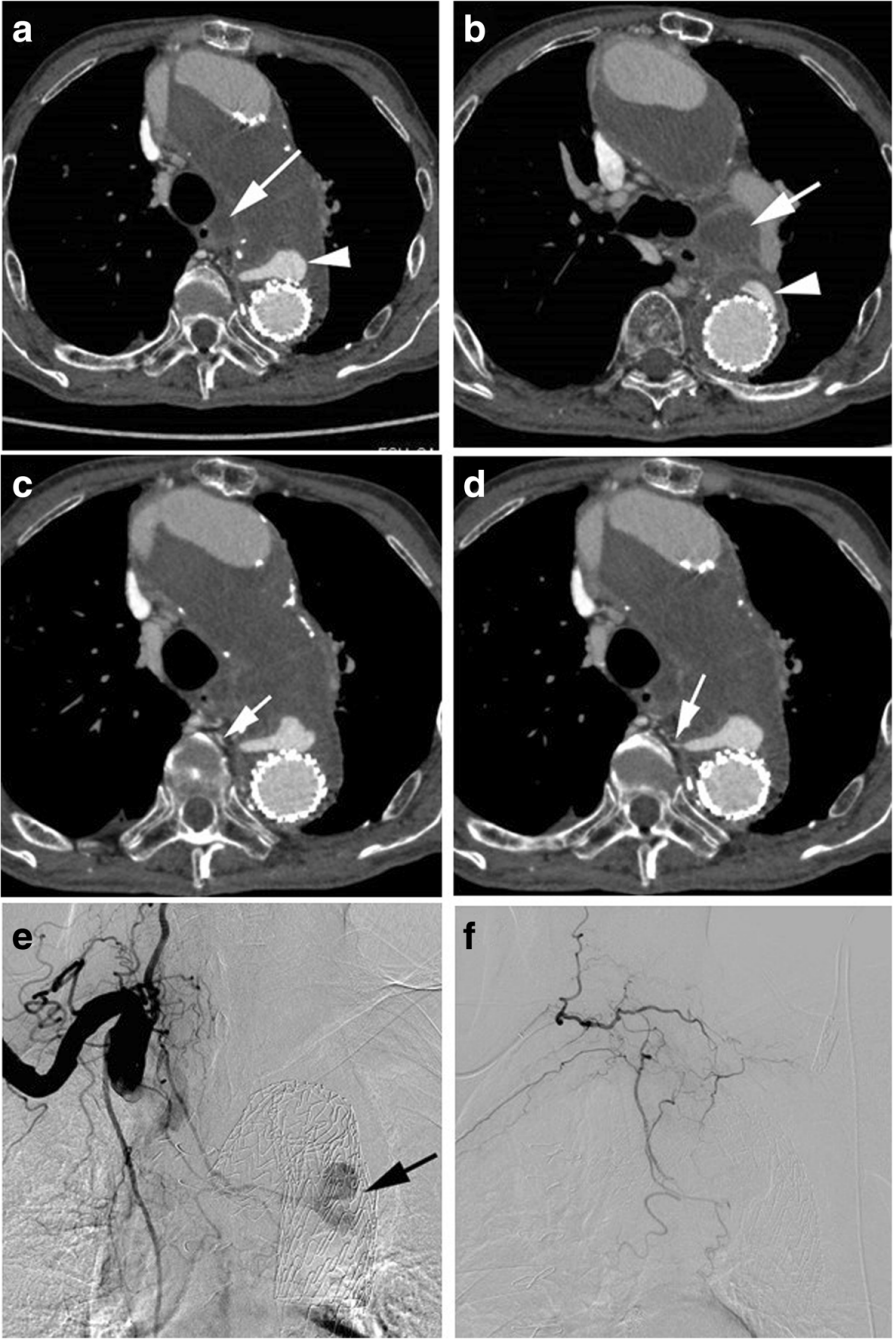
a and b. Two stent-grafts were inserted into the region extending from the aortic arch (elephant trunk) to the descending aorta. CT showed a type 2 endoleak. The pseudoaneurysms remained (arrows). c and d. CT showed a type 2 endoleak via the bronchial artery (arrows). e. Brachiocephalic arteriography showed a type 2 endoleak (arrow) into the aortic pseudoaneurysms from the supreme intercostal artery via the bronchial artery. f. After TAE, the type 2 endoleak disappeared
